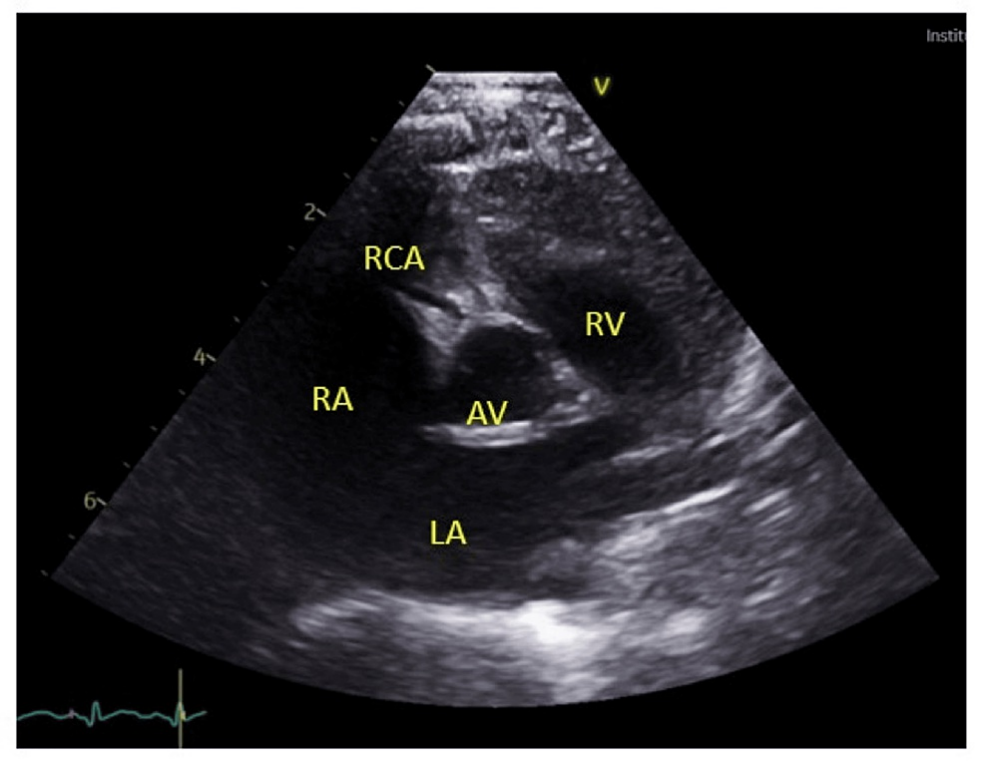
An echocardiogram (ECHO) showing normal-size RCA RCA: right coronary artery, RA: right atrium, LA: left atrium, RV: right ventricle, AV: aortic valve
